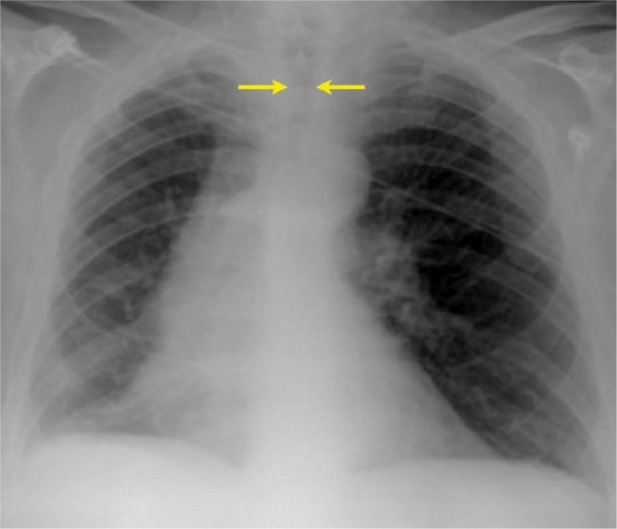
Chest radiograph is apparent for luminal narrowing of the trachea.
